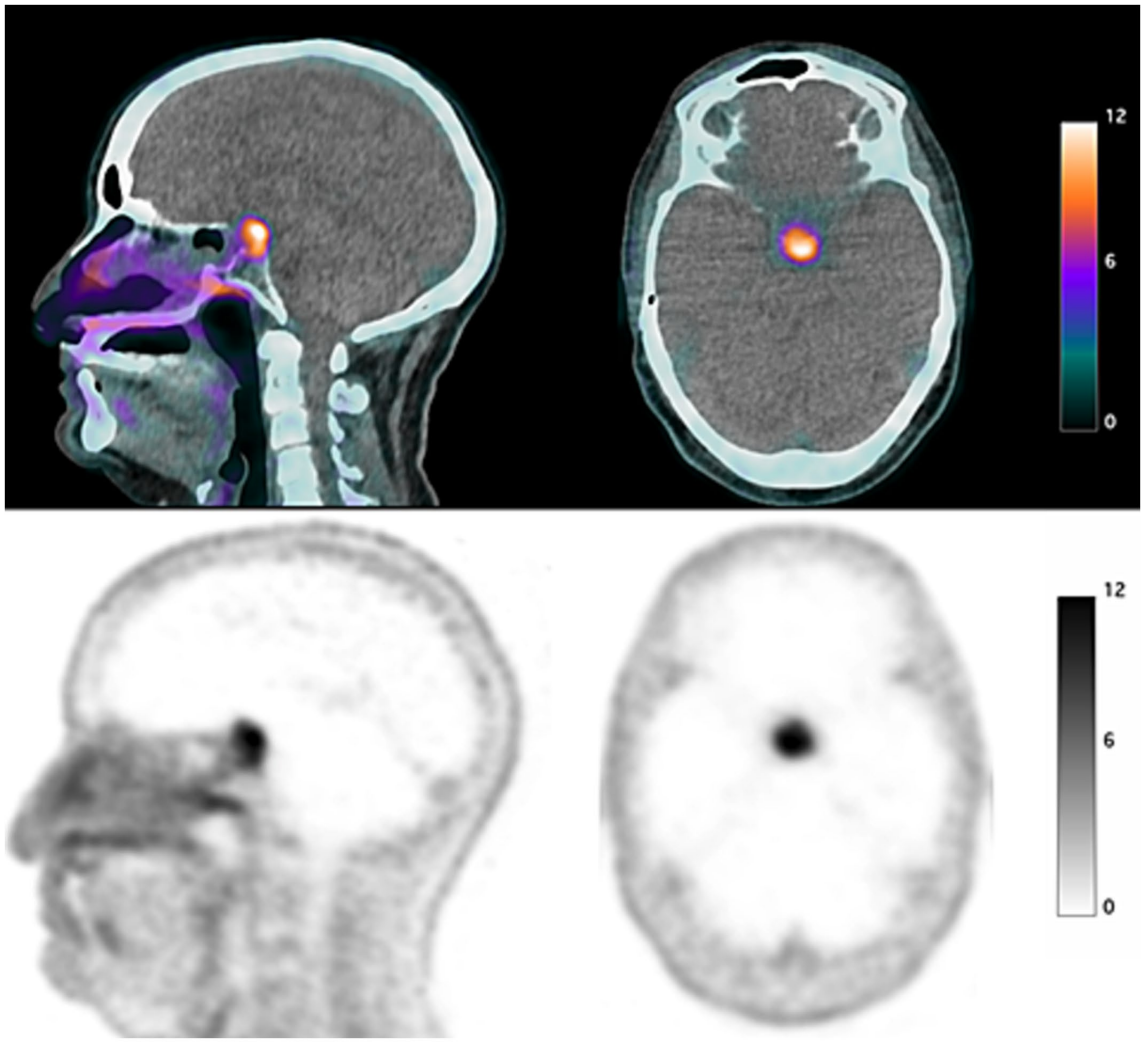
PET/CT showing an intense unhabitual fixation centered on the sellar region. Upper part: Fusion PET/CT axial and coronal; Lower part: PET axial and coronal.
